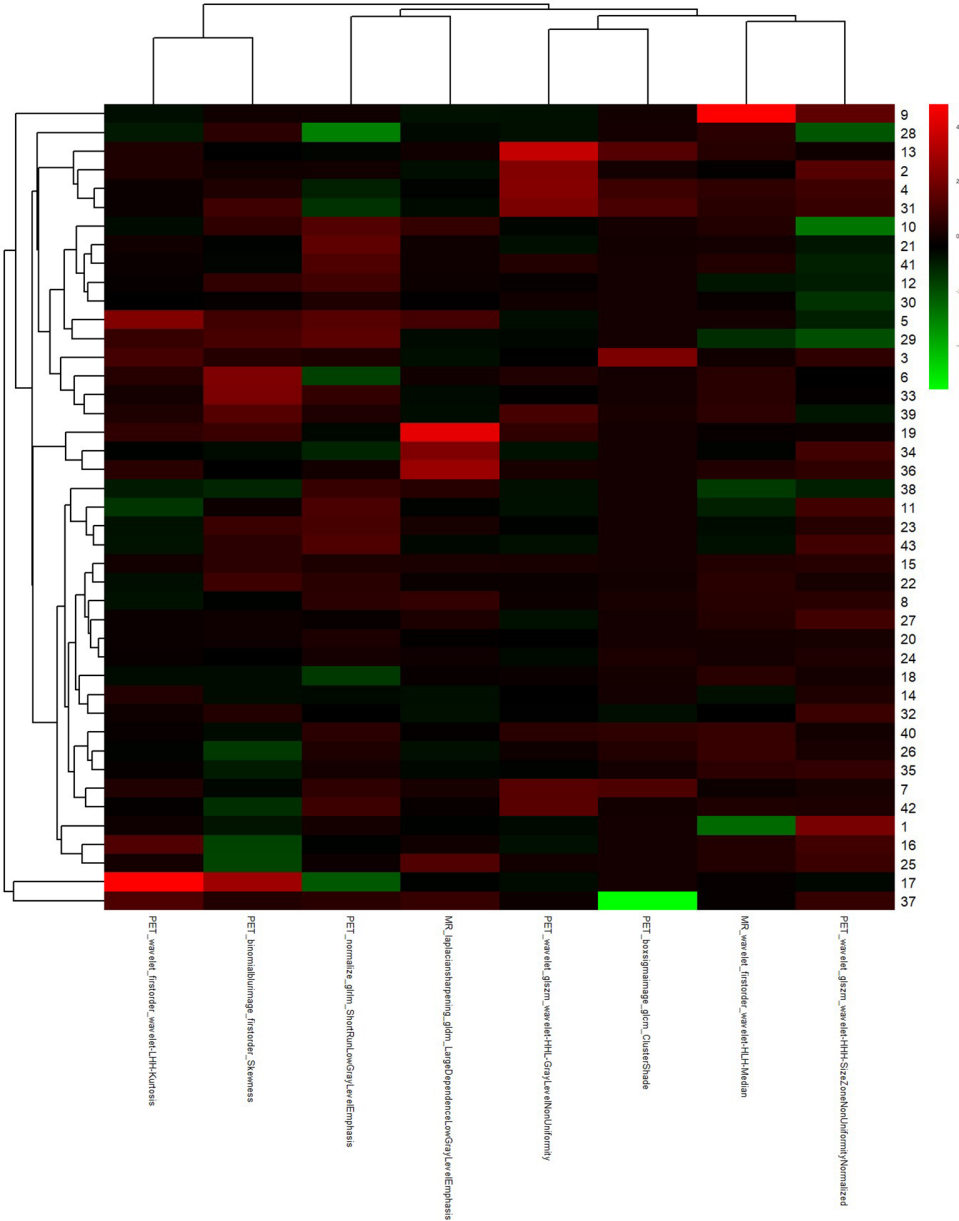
The most predictive feature subset of PET/MRI.
